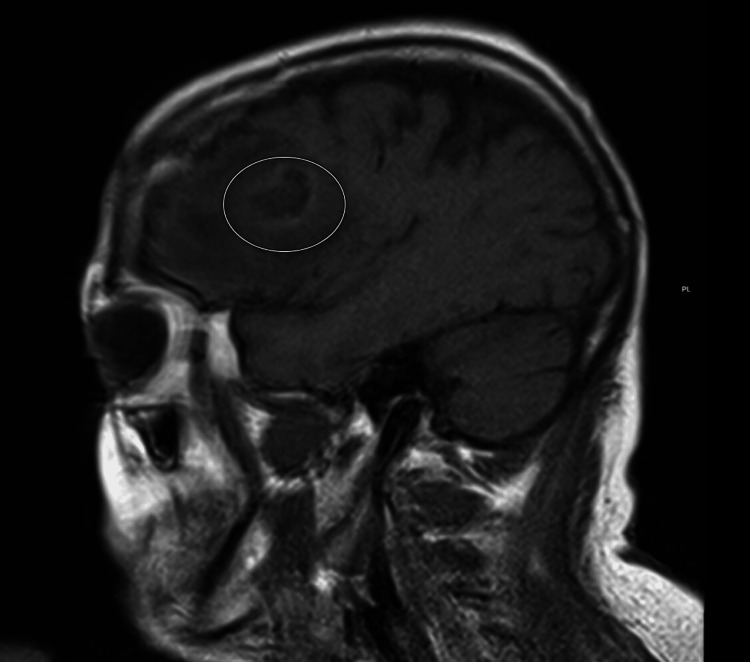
T1-weighted sagittal magnetic resonance imaging (MRI) of the head with contrast, showing a rim-enhancing mass in the right frontal lobe without involvement of the maxillary sinus.
